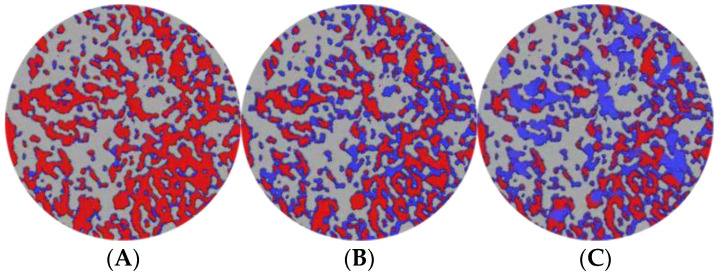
Distribution maps generated at different displacement stages for the remaining oil samples using the CT scanning technique in the 2D plane. (A) Initial; (B) water flooding; (C) heavy oil activator flooding.
